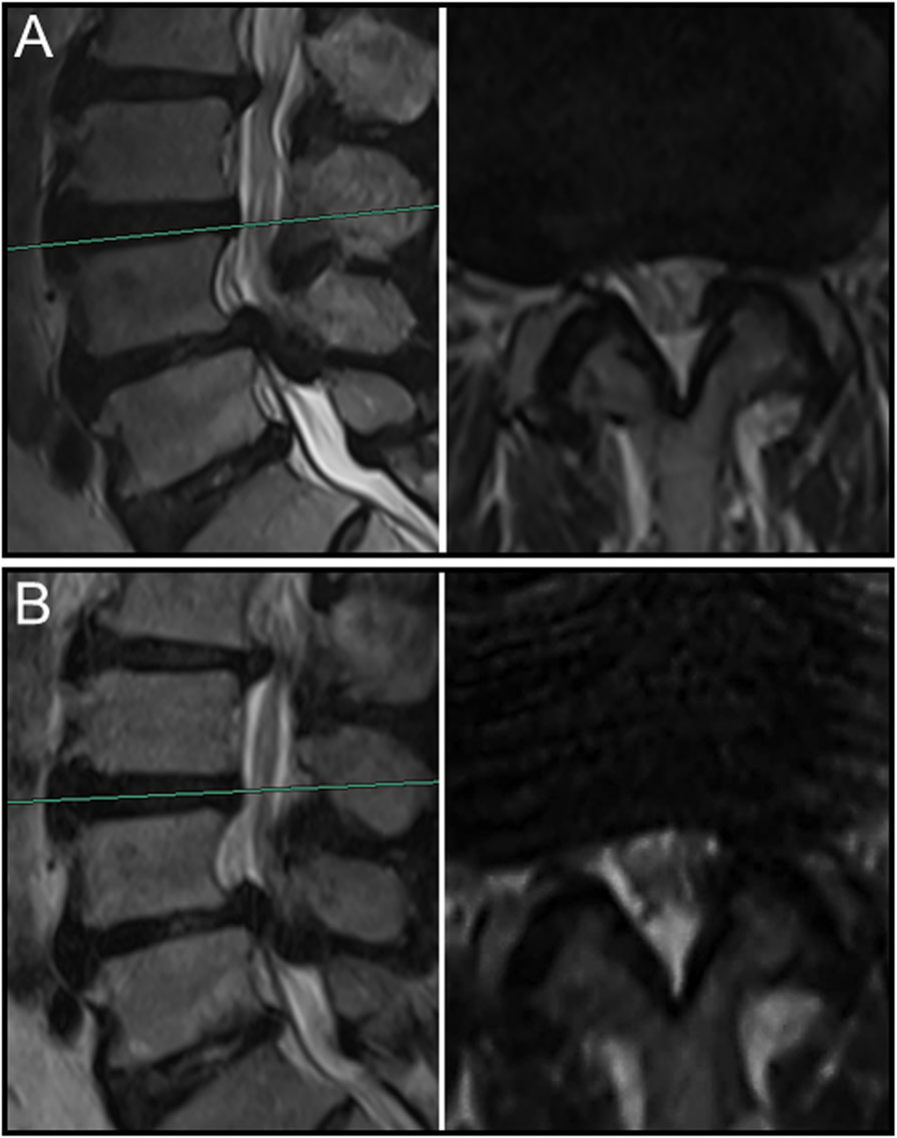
In a man with positive SedSign and multiple level spinal stenosis (a), the nerve roots remained stay the ventral side of dural sac on prone MR images (b)
